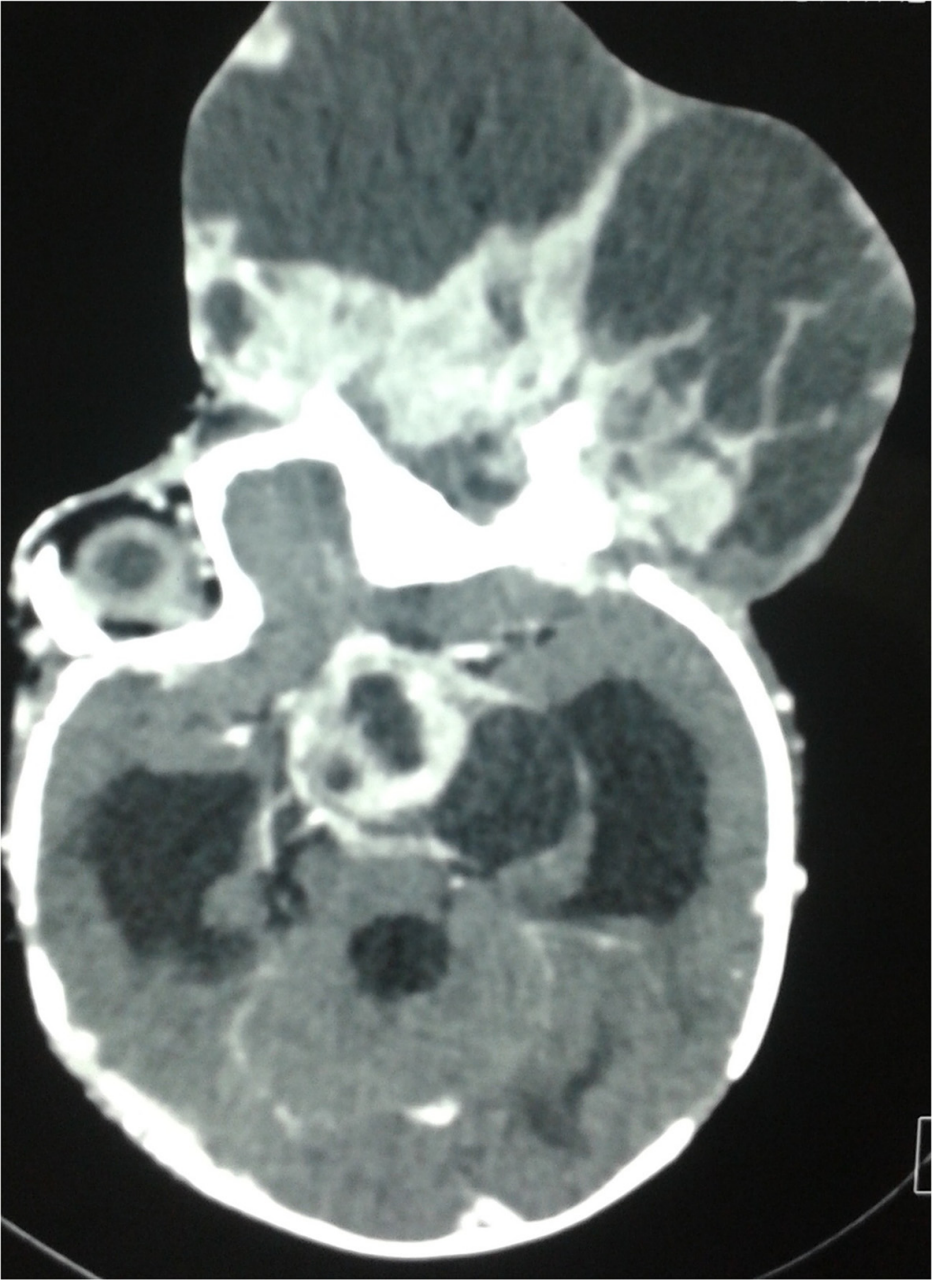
Evolution after 2 courses of chemotherapy. Axial T2-weighted MR image redone objectives a huge heterogeneous orbito-cerebral mass, enhanced intensely after injection of contrast agent and associated to triventricular dilatation.
